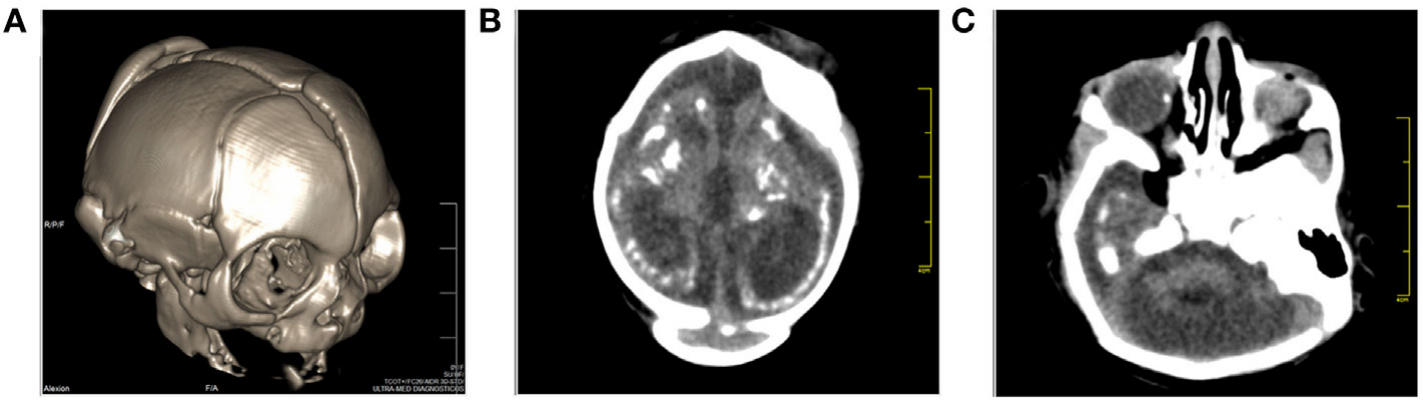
Cranial computed tomography (CT) images of the baby born after Zika virus infection in pregnancy. (A) Sagittal localizer CT image of the markedly abnormal skull shape, (B) axial CT images showing microcephaly, cerebral atrophy, and multiple dense intracranial periventricular calcifications located in the subcortical white matter at the gray matter–white matter interface, and (C) ocular calcification.
